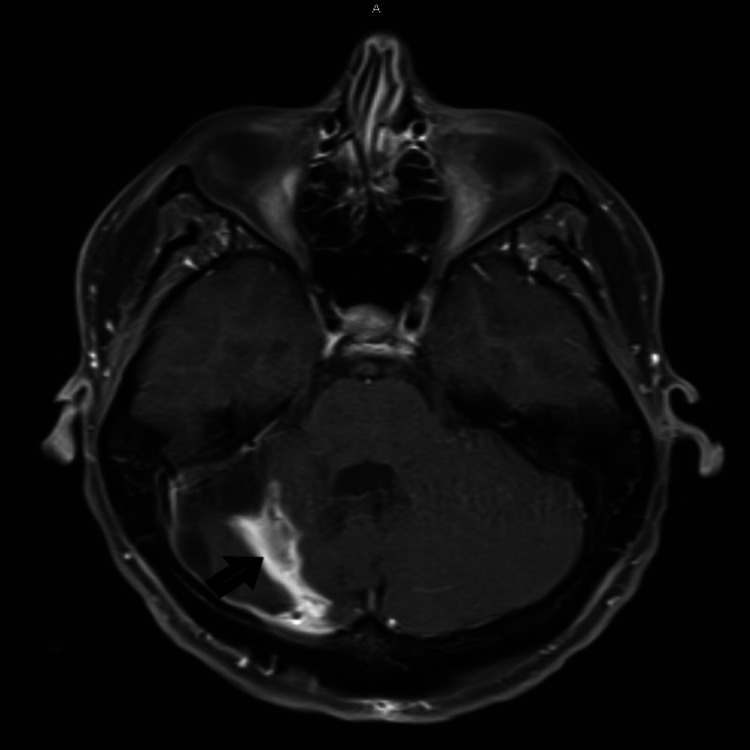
MRI of the brain showing finding suggestive of postoperative changes to the right cerebellum.
